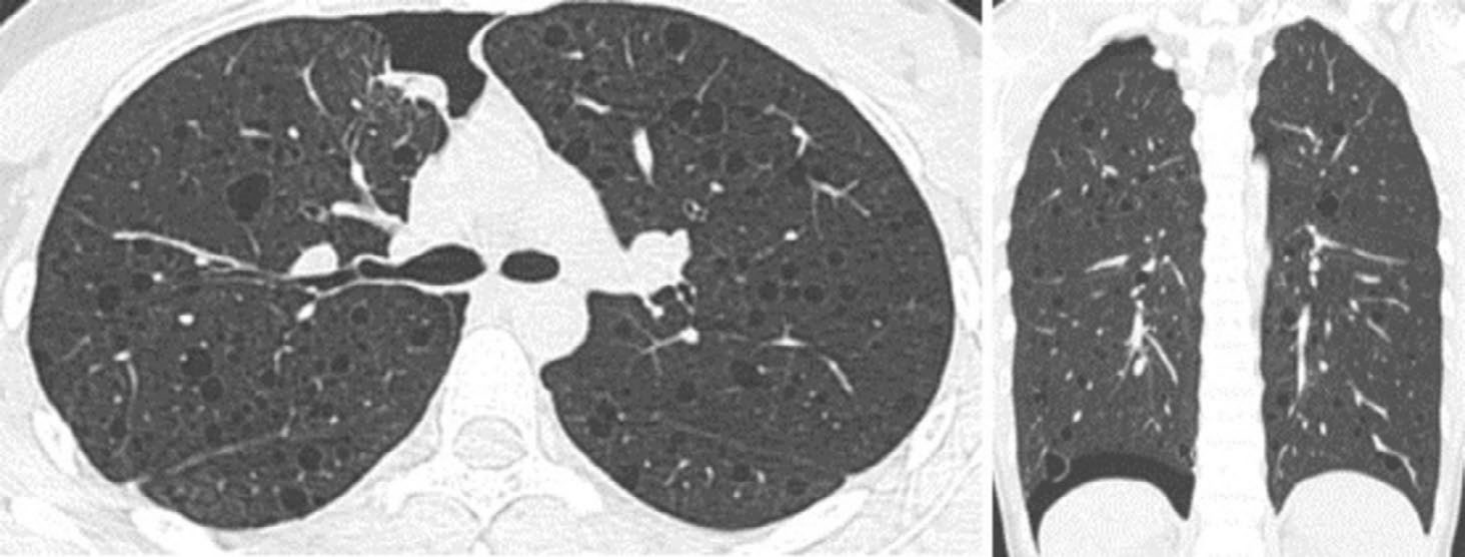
Chest CT scan performed on May 2 shows a significant reduction in the right pneumothorax, with residual lung compression of approximately 5%. There is complete resolution of the left pneumothorax and marked improvement in right anterior chest wall subcutaneous emphysema.
